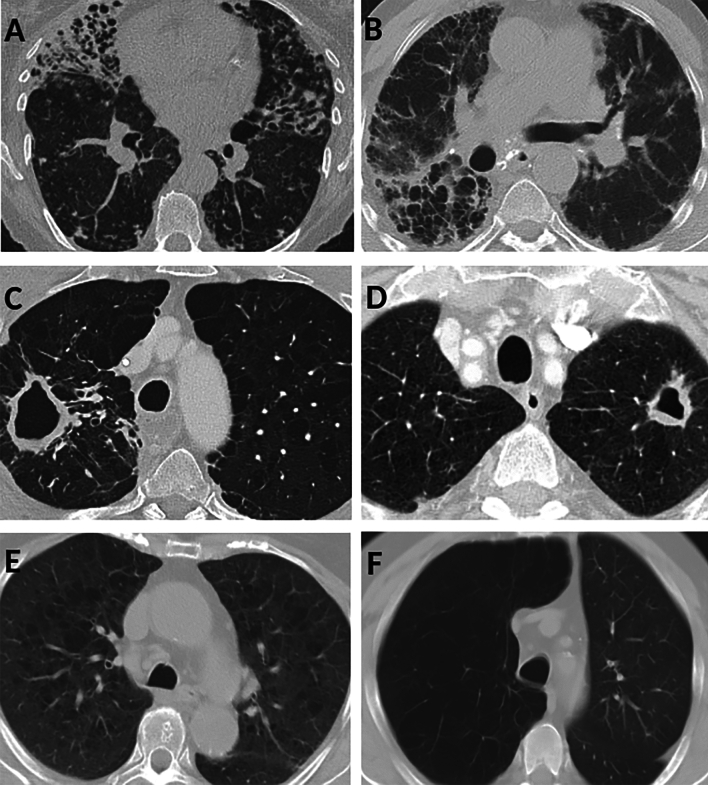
Exemplary CT images of included patients with SGM: high grade bronchiectasis in two patients with *M. intracellulare* (A,B), cavity and emphysema in a patient with *M. chimaera* (C) and with *M. avium* (D), emphysema in a patient with *M.*
